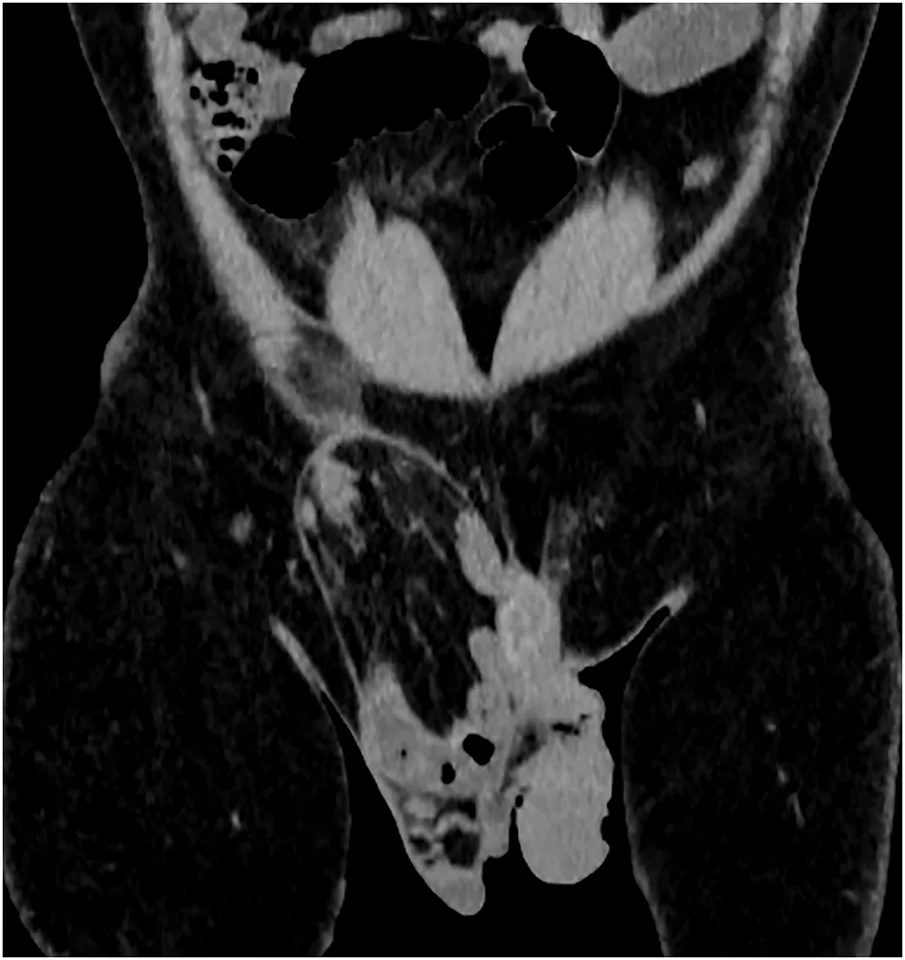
CT revealed a right indirect inguinal hernia with the small bowel prolapsing into the scrotum.
